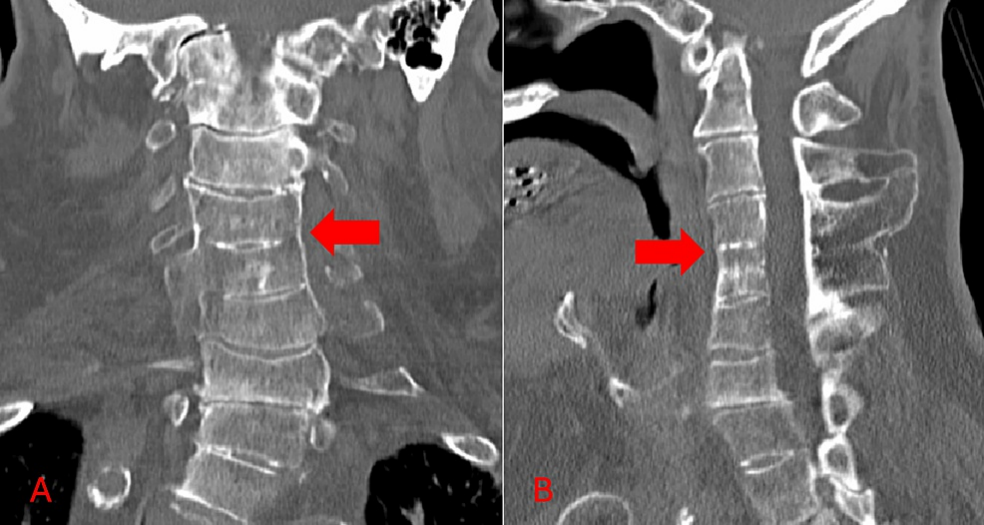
Coronal (A) and sagittal (B) CT scans of the cervical spine in Klippel-Feil syndrome. CT scans were conducted without contrast. Fusion between C3-C4 and deformities affecting several cervical vertebrae are evident. Red arrows in both panels highlight the fusion.
